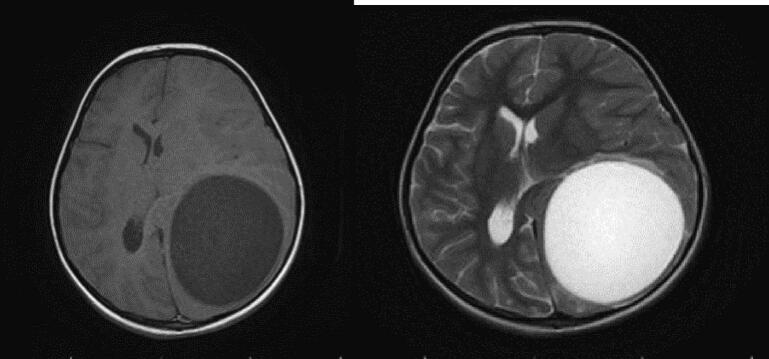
Axial T1W and T2W MRI showing a spherical and well-defined, smooth, thin walled, homogeneous cystic lesion with signal similar to the cerebrospinal fluid without septations.
